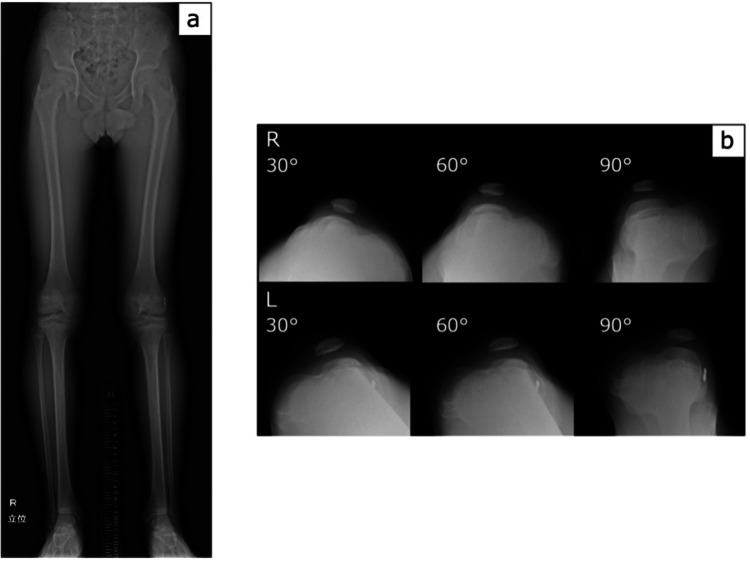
Standing radiograph and skyline images of the patella at 12 years of age Full-length radiographs of the standing lower limb (a) and skyline images of the patella (b) obtained before surgery on the right knee at 12 years of age.
